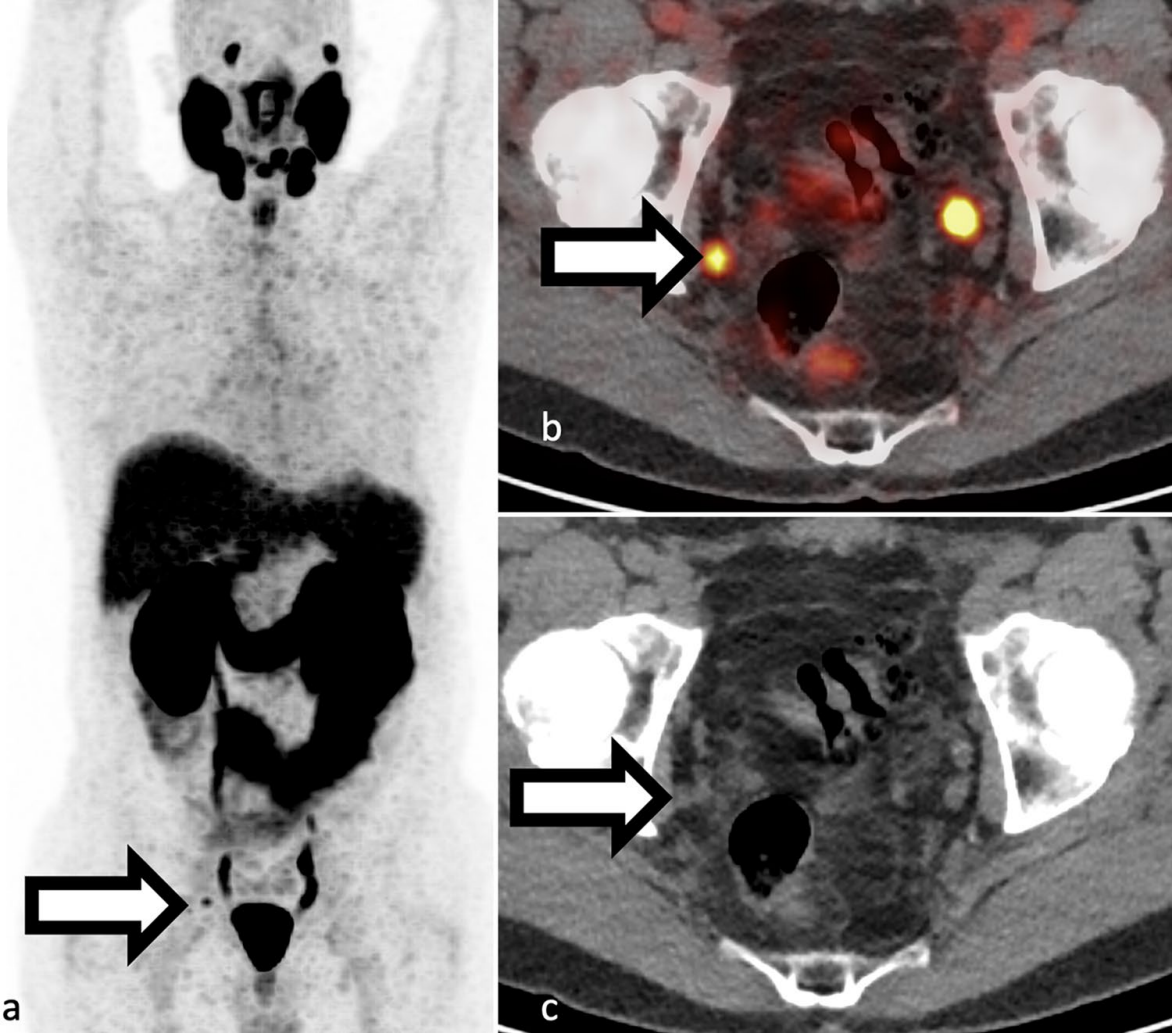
a–c Example of single PSMA lymph node disease in a 54-year-old with recent resection for prostate cancer with persistently elevated PSA. F18-DCFPyL PET/CT showed a single focus of PSMA uptake in right pelvis (panel a and b, SUVmax 4.4) corresponding to a 4 mm right pelvic side wall lymph node (panel c). Original preprostatectomy pathology showed up to Gleason 4+5 prostate adenocarcinoma in left prostate lobe and 10/17 cores were positive for cancer. Uptake in the left pelvis corresponds to ureteric activity. Maximum intensity projection view a SUV scaled at 0–5
